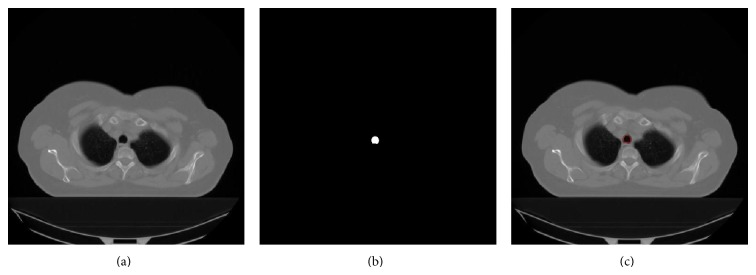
Segmentation of the trachea/main bronchi: (a) original CT slice, (b) segmented trachea/main bronchi in white, and (c) trachea/main bronchi contour in red superimposed on the original slice.
